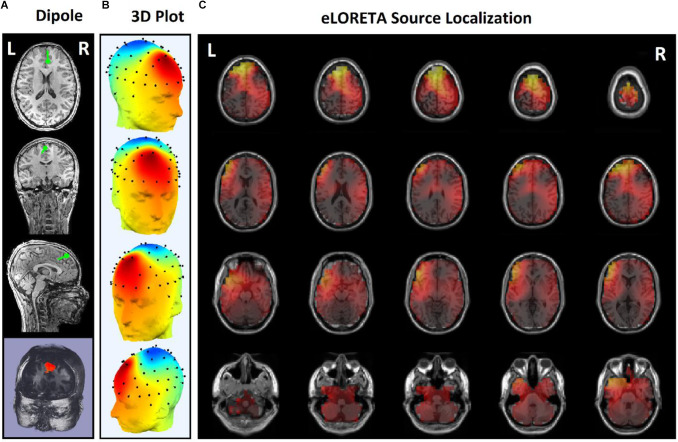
Source localization of the rTMS stimulation foci in center of frontal for a responder subject. (A) Dipole coordinates of the identified focus in the center of frontal region on a default MRI. (B) Position of the foci identified by ICA on the surface of the skull. (C) Source localization in right DLPFC by eLORETA.
